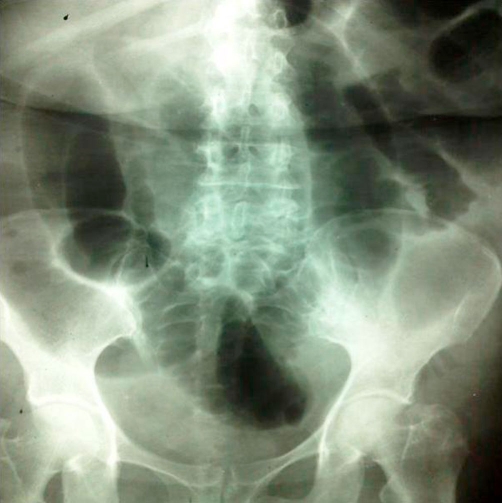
Abdominal X-Ray in favor of bowel obstruction.
